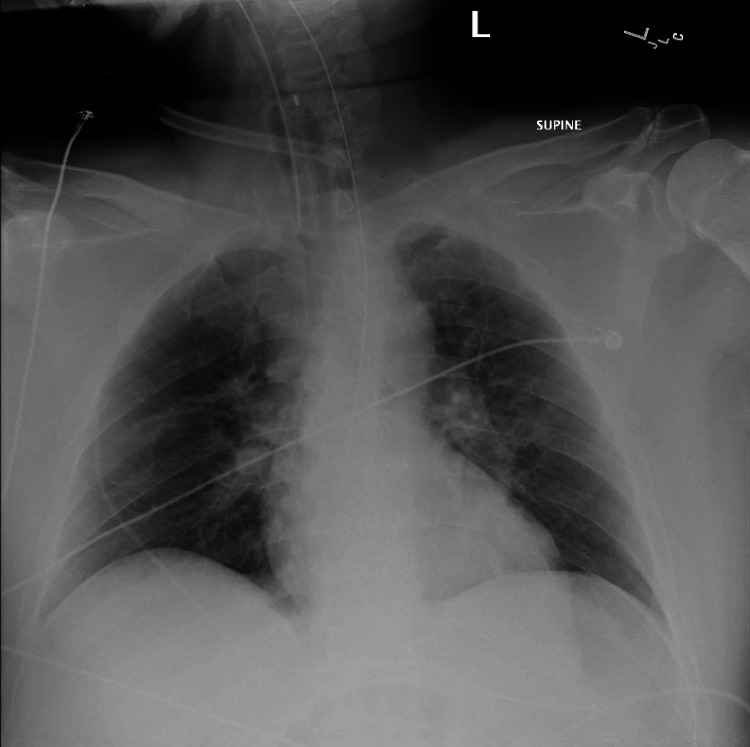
Post-event chest X-ray showing hilar fullness, likely from early pulmonary edema
